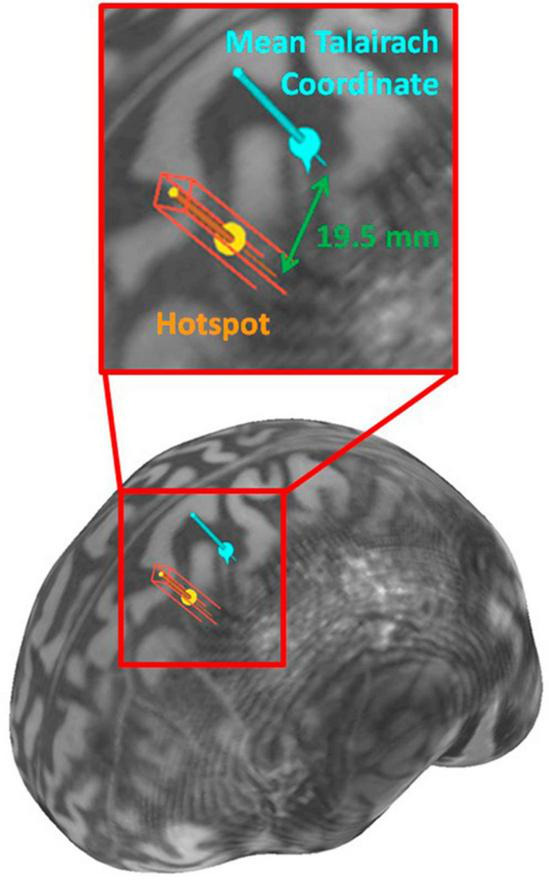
Brain surface visualization rendered from Participant 1’s MRI with the hotspot and the mean Talairach coordinate.
